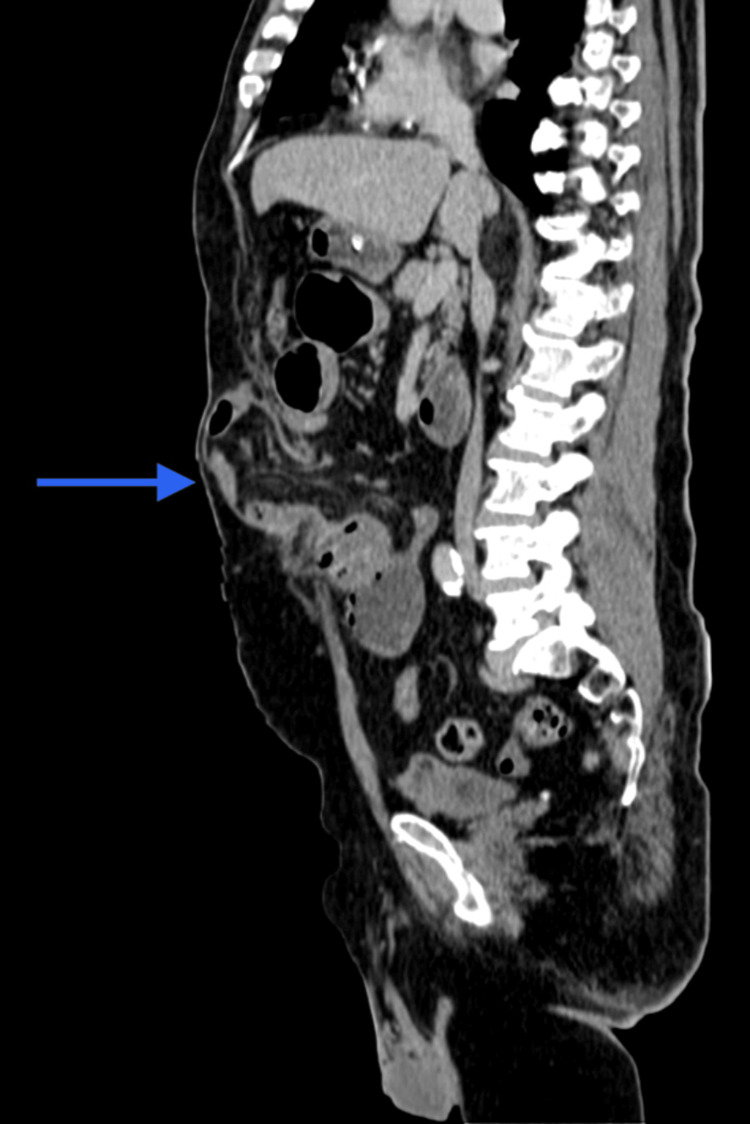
CECT suggests an anterior abdominal wall defect of size 4.6 x 4.8 cm at the level of L1-L2. CECT: Contract-enhanced computed tomography
